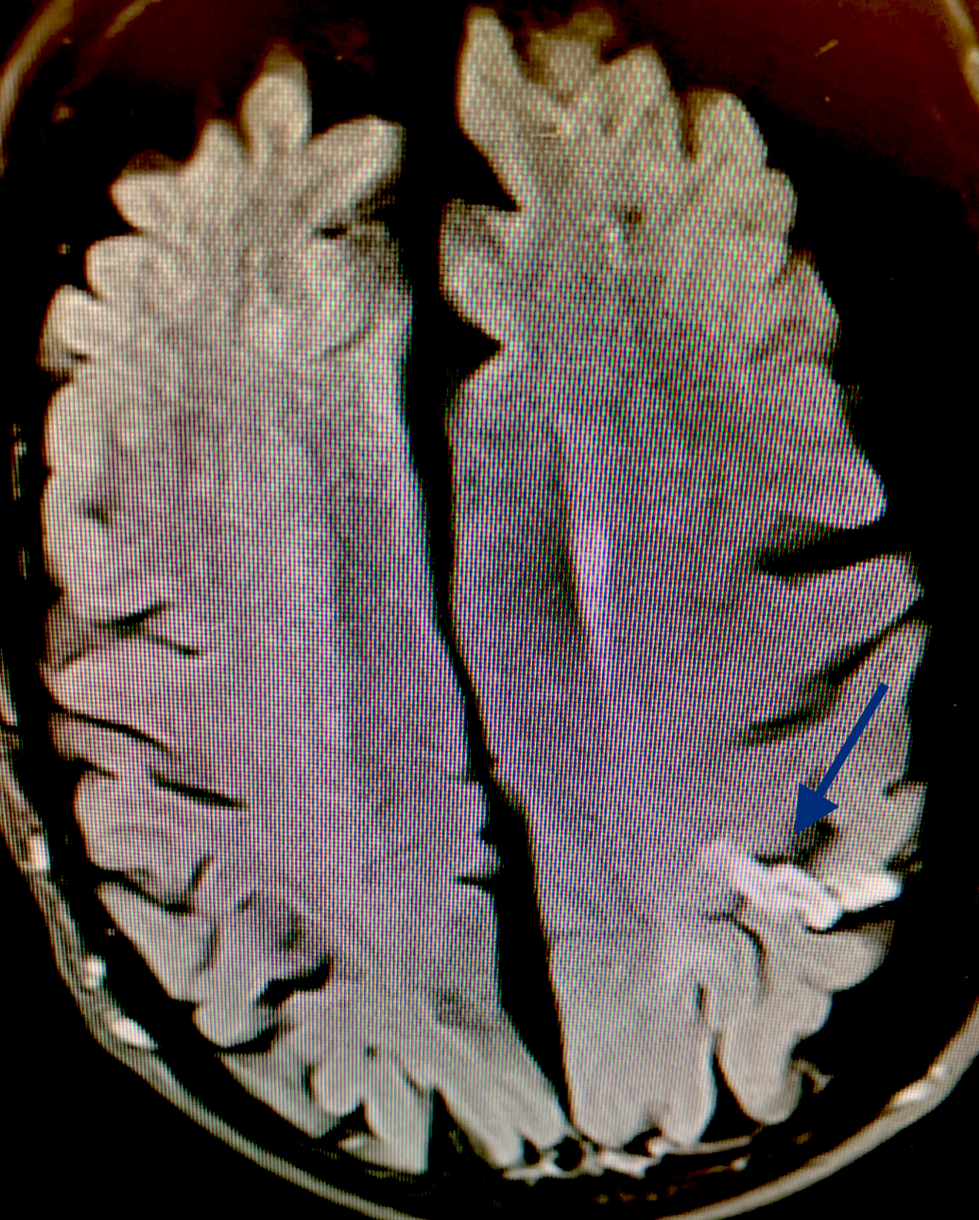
MRI-DWI showing left posterior parietal lobe cortical ischemic infarct (blue arrow) MRI: magnetic resonance imaging; DWI: diffusion-weighted imaging
